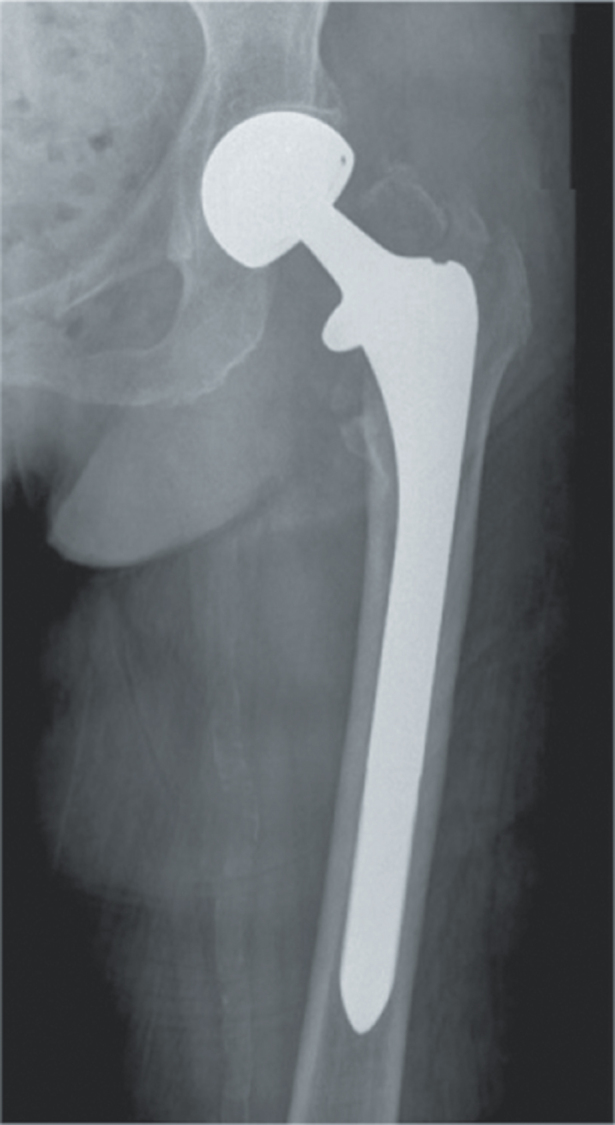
X-Ray views of the long-stem cementless bipolar hemiarthroplasty (LSCBH).
